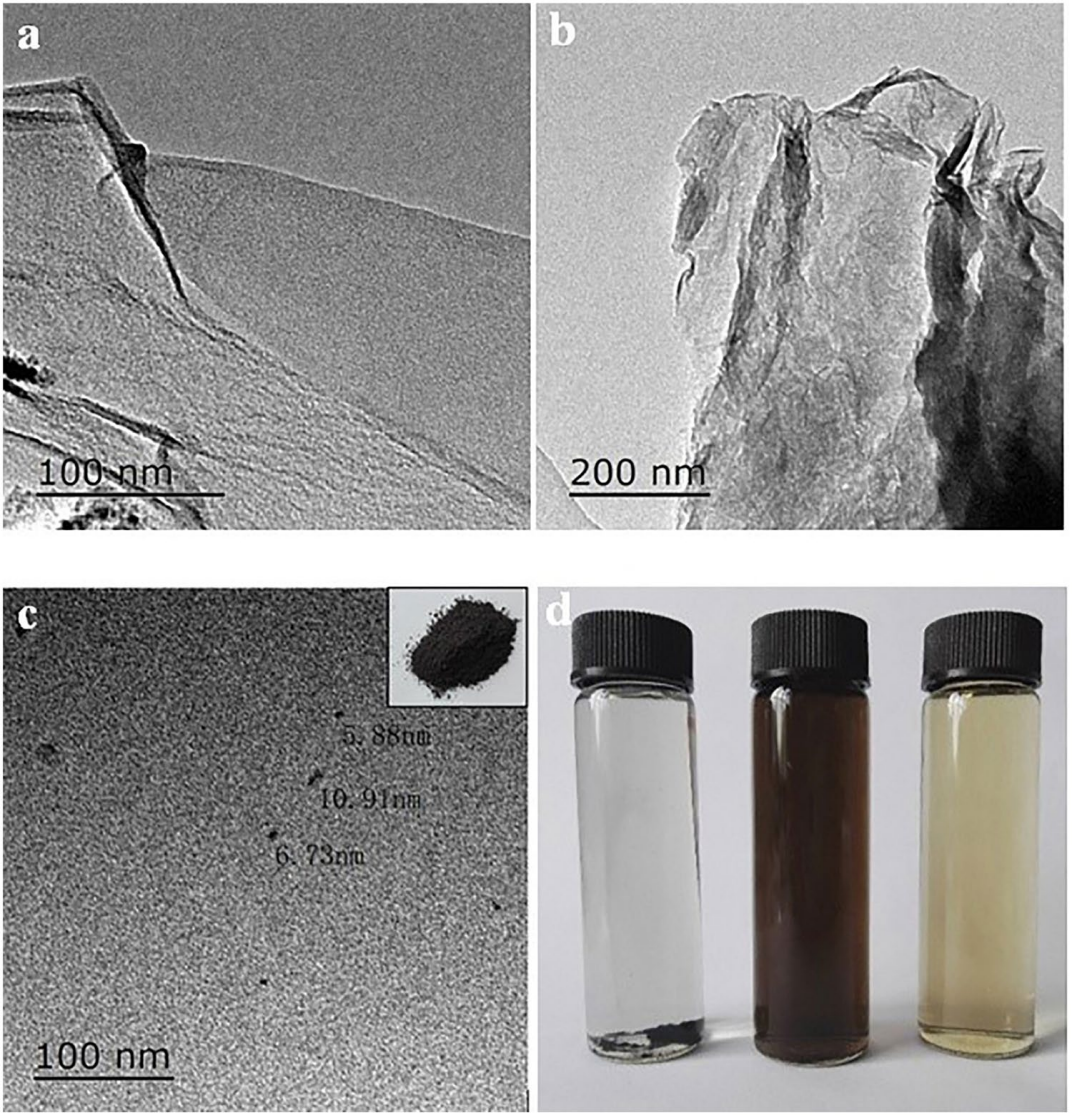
TEM images of (a) GO, (b) RGO, and (c) GQDs (inset image is GQDs powder). (d) their water-dispersing behavior (1 mg/mL) after an ultrasonic treatment for 30 days (left: RGO, middle: GO, right: GQDs).
